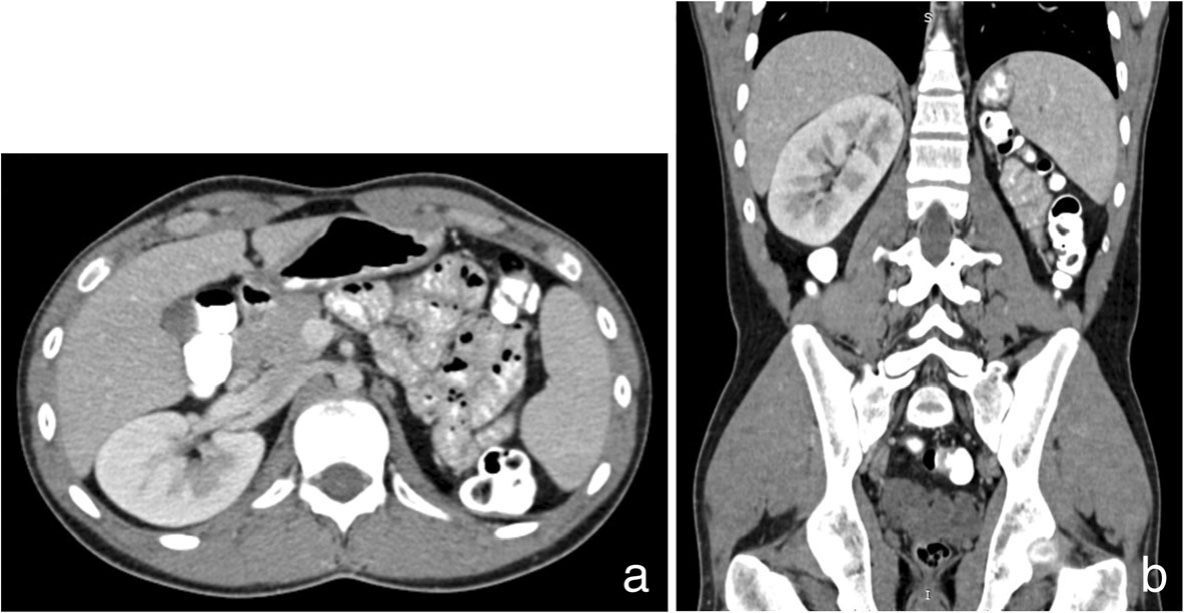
Contrast-enhanced axial (a) and coronal reconstruction (b) CT images of the abdomen reveal only a right kidney with absence of a left kidney
